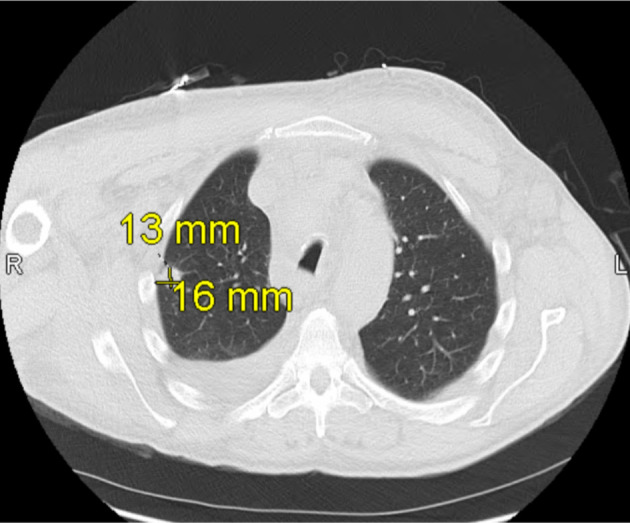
Computed tomography (CT) scan of the chest obtained on hospital day 2 shows a 13 × 16 mm mass in the lateral right upper lobe with irregular margins and extension to the pleura, consistent with findings during early hospitalization for suspected superior vena cava (SVC) syndrome.
